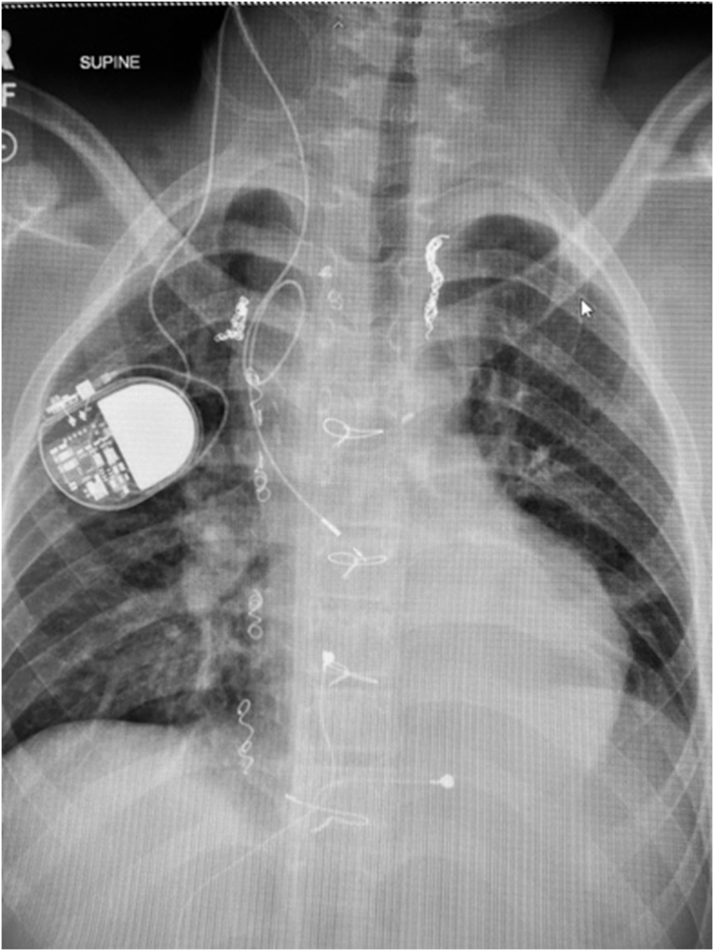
Final Chest X-Ray Anterior-posterior chest x-ray demonstrates transvenous transpulmonary atrial pacing lead placed through the right internal jugular vein and superior vena cava onto the epicardial common atrium with generator overlying right upper chest.
